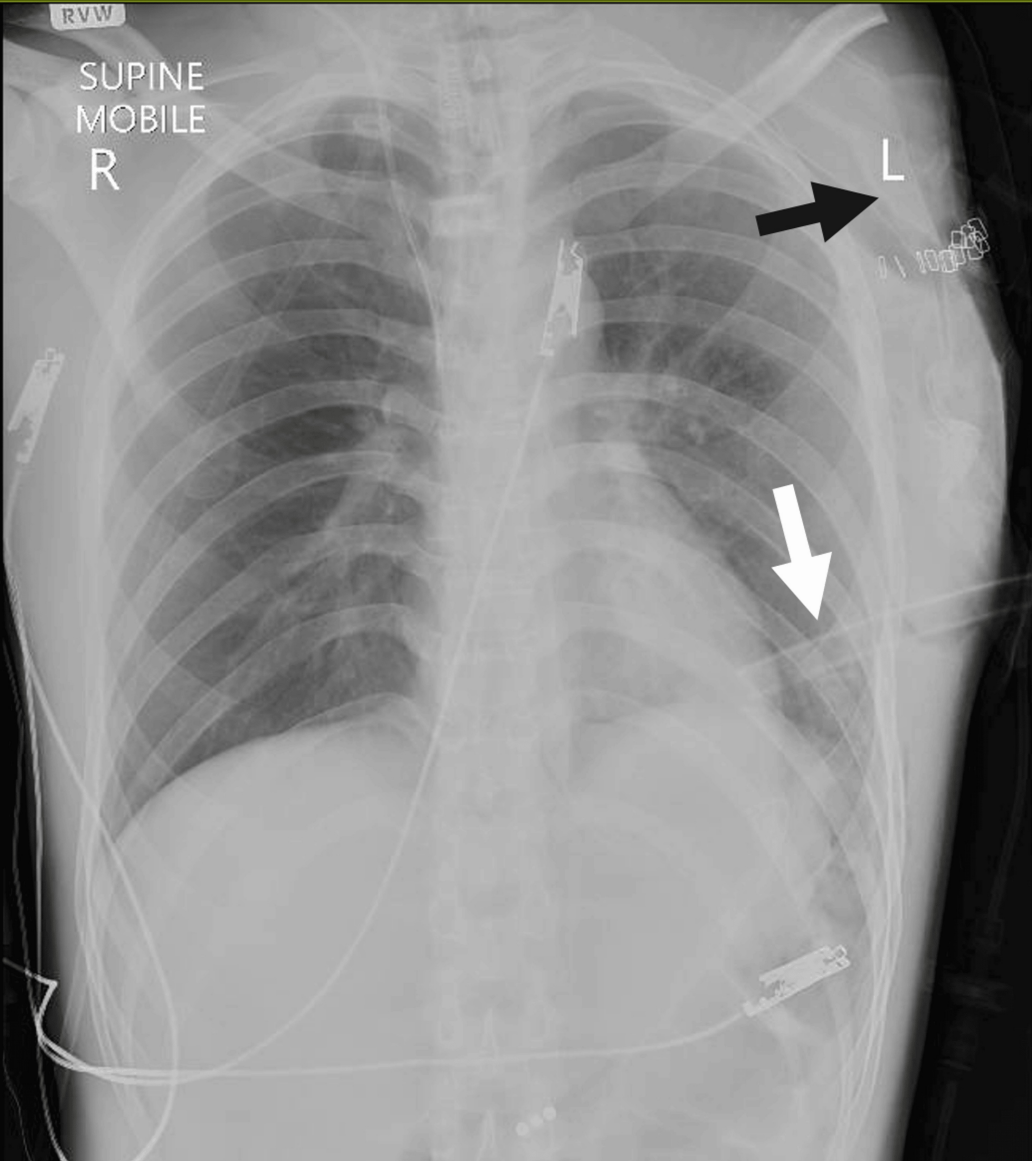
Postoperative chest radiograph White arrow: The intercostal drain position and the absence of immediate thoracic complications following forequarter amputation; Black arrow: Skin staples at the stump site
